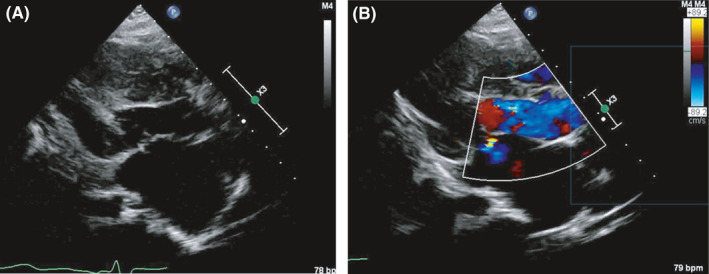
Postoperative transthoracic echocardiography images. A, The parasternal short‐axis view shows improvement in the left ventricular outflow tract obstruction and systolic anterior motion. B, A Color Doppler shows only a trace mitral regurgitation
